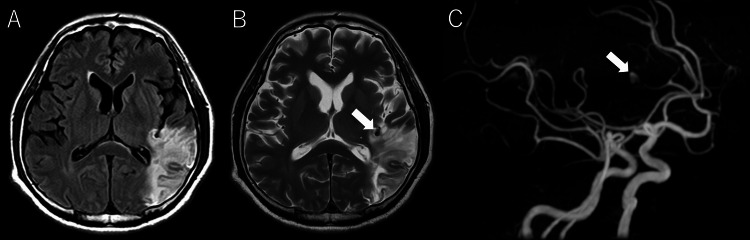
Post-mitral valve repair head MRI/MRA. (A) FLAIR image; (B) T2-weighted image; and (C) MRA. Chronic cerebral infarcts are observed, along with an aneurysm in the left middle cerebral artery (arrow). MRA: Magnetic Resonance Angiography; FLAIR: Fluid-Attenuated Inversion Recovery image.
